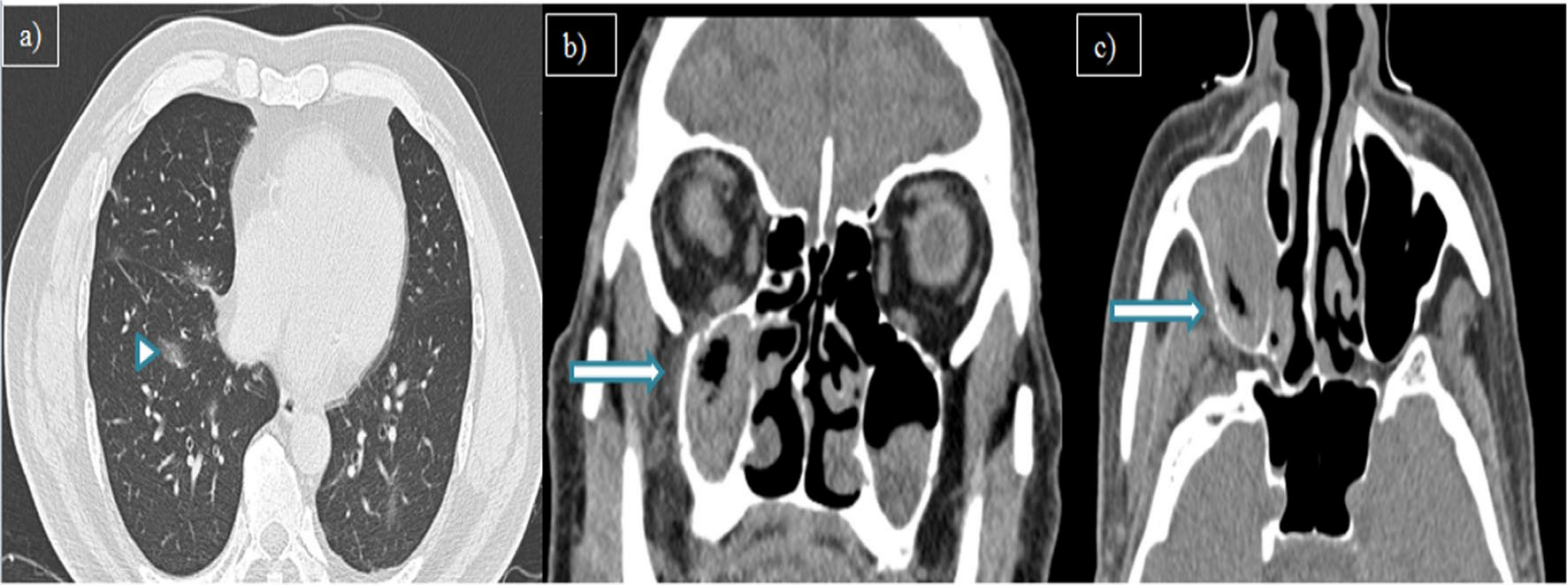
a Axial HRCT thorax, b Coronal, and c Axial CT paranasal sinus images of a 44-year-old male patient with complaints of breathlessness and rhinorrhea showing patchy ground glass opacities in right lower lobe (arrowhead) and bilateral maxillary sinusitis with periantral space fat stranding (arrow)
